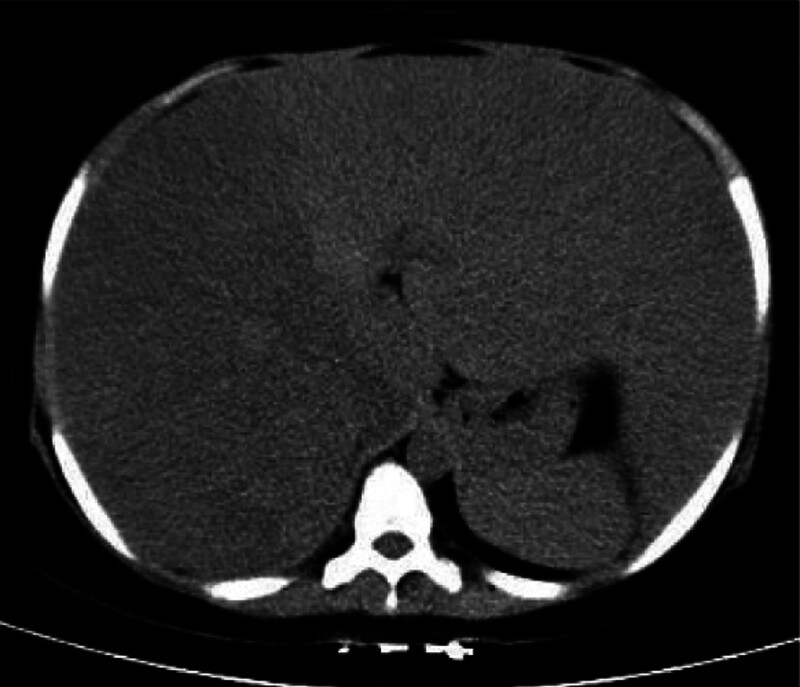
A plain CT scan of the upper abdomen. The liver is obviously enlarged, its density is obviously uneven, and the mosaic-like appearance is seen. The density of the right lobe is slightly lower than that of the left lobe. CT = computed tomography.
